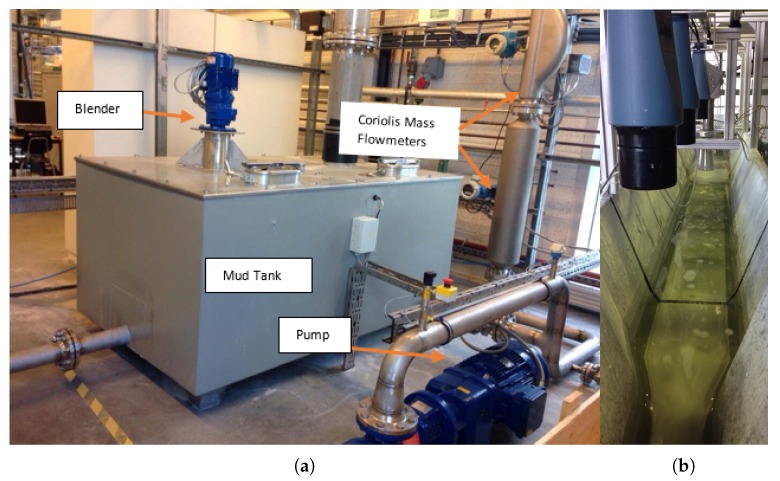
(a) Test flow loop at University College of Southeast Norway, Kjølnes Campus, showing mud tank, blender, pump and Coriolis flowmeters. (b) Open Venturi channel with ultrasonic level sensors.
